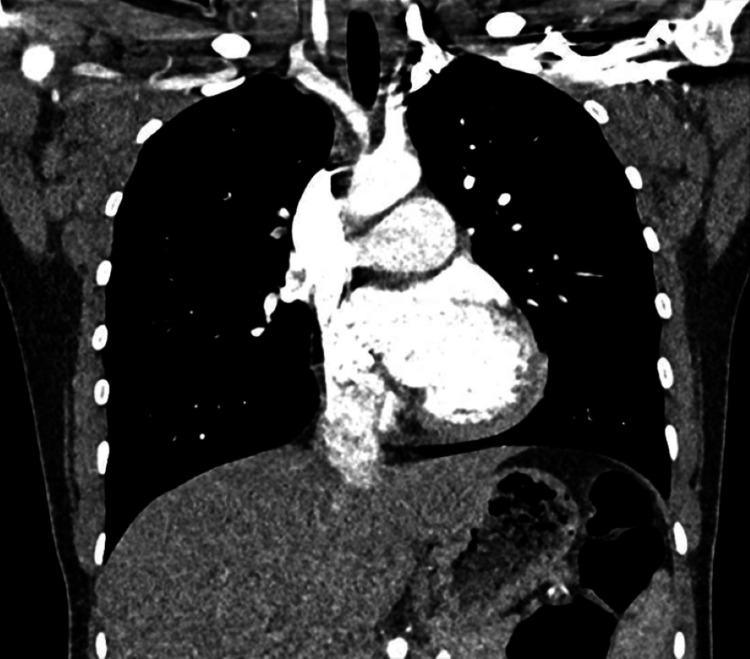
Coronal CT of the chest. A coronal contrast CT of the thorax demonstrating bilateral axillary lymphadenopathy in a patient later confirmed to have Kikuchi-Fujimoto disease. The enlarged lymph nodes are well-defined, discrete, and non-necrotic in appearance, with no mediastinal or hilar involvement.
